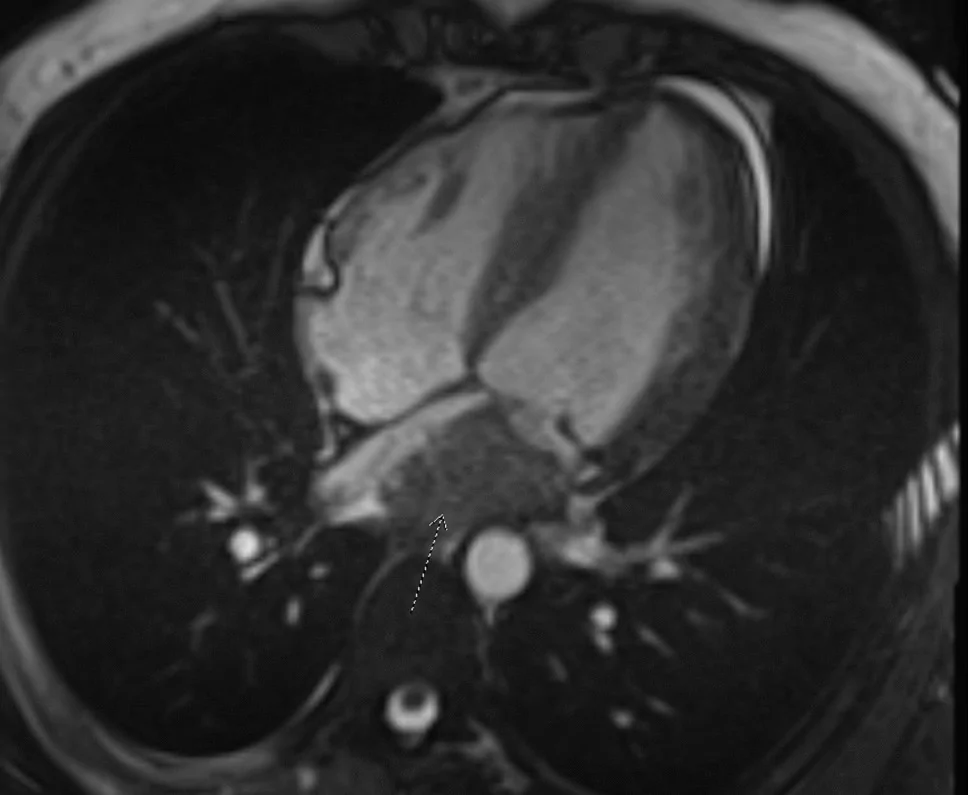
Preoperative cardiac MRI T2 sequence demonstrates a hypointense lesion filling the majority of the left atrium as well as apical pericardial effusion
